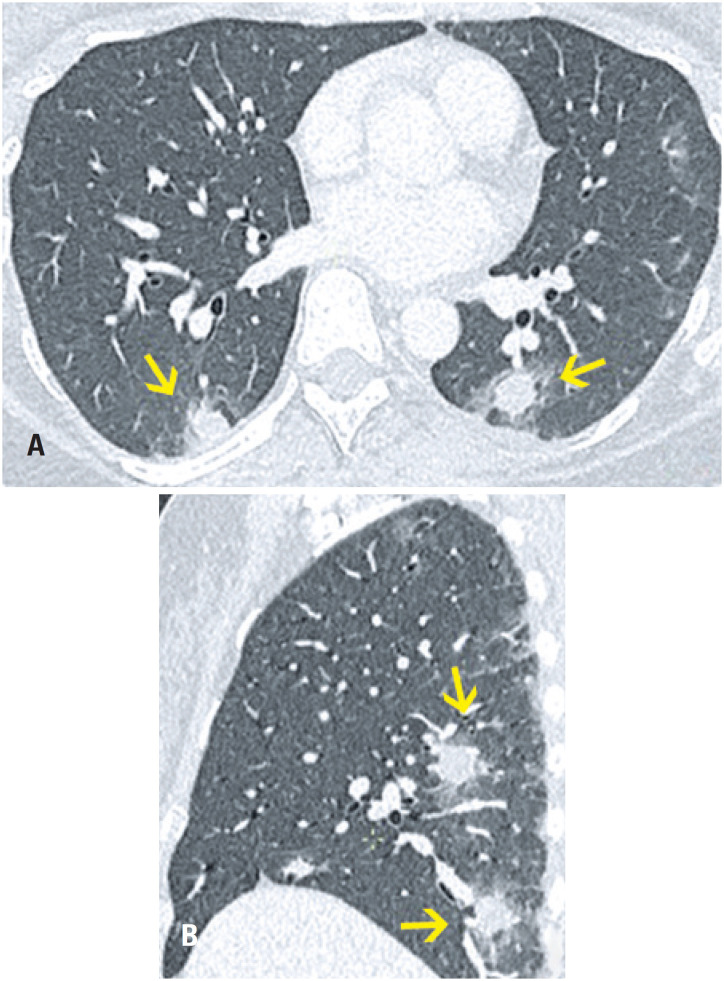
A 39-year-old female patient with cough. Computed tomography (A) Axial section (B) Sagittal reformation image: multiple pulmonary nodules with a ground-glass halo spread through the lungs, larger and more numerous in the middle and lower fields. Confirmed using measles serology
